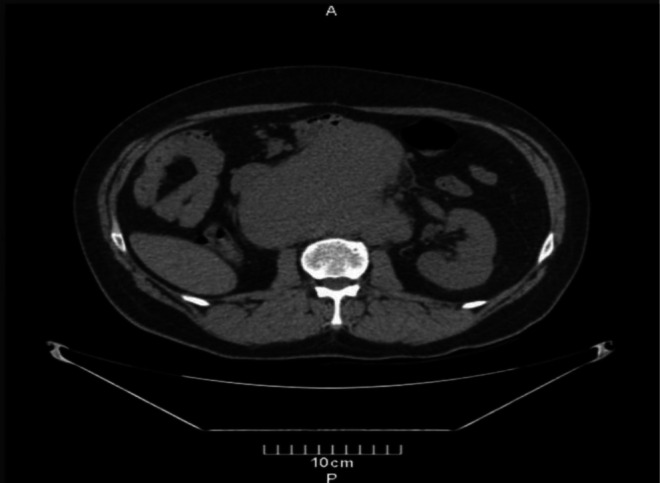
CT scan of abdomen, showing a retroperitoneal mass, suggestive of lymphadenopathy.
